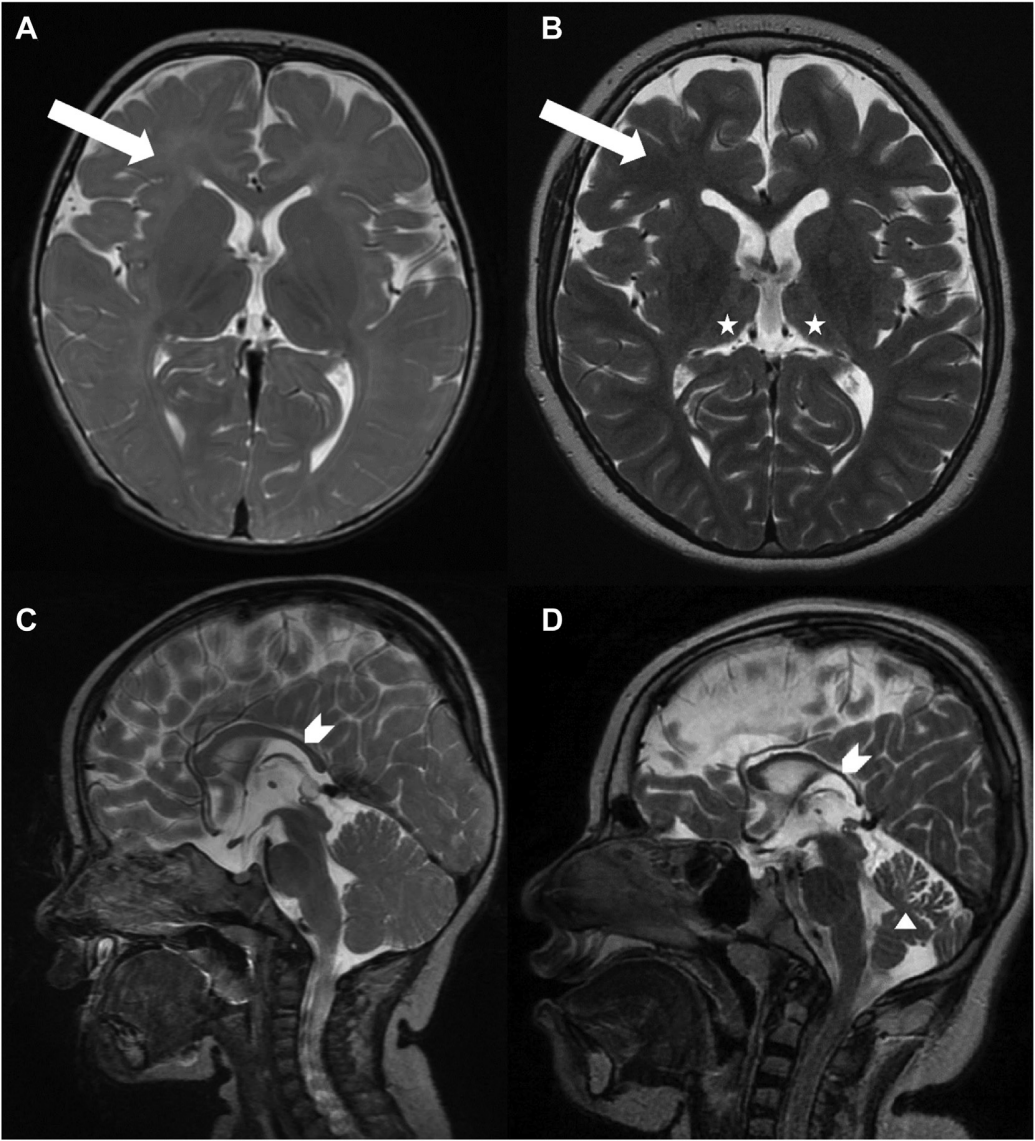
Sequential brain MRI of patient 1: Axial T2-weighted image at the age of 11 months (A) and 11 years (B) respectively showing insufficient myelination (A) (arrow), progressive myelination (B) (arrow) and atrophic thalami (B) (asterisk). Sagittal T2-weighted image at the age of 11 months (C) and 11 years (D) respectively with thinning of the corpus callosum (arrowhead) and cerebellar atrophy (triangle).
